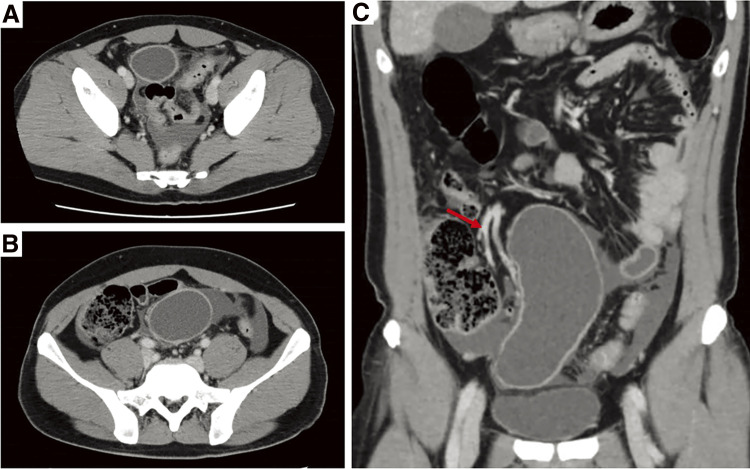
Preoperative contrast-enhancement CT images (Case 1). Axial views of CT scans showed a cystic lesion with well contrast-enhanced wall, which has no communication with the intestinal tract (A, B). In addition, a moderate amount of ascites was evident around the cystic lesion and in the Douglas pouch. Coronal sections depicted the ileocolic artery (red arrow) as a main feeder of the cystic lesion (C).
